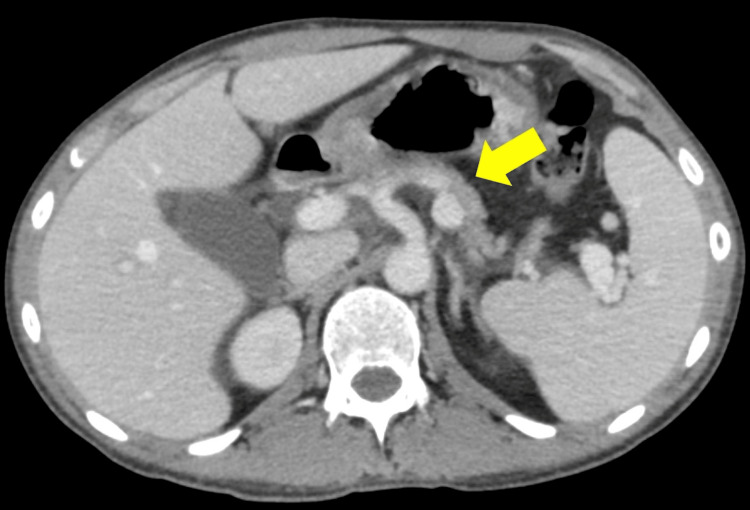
Abdominal plain computed tomography scan The yellow arrow indicates an atrophic pancreas.
